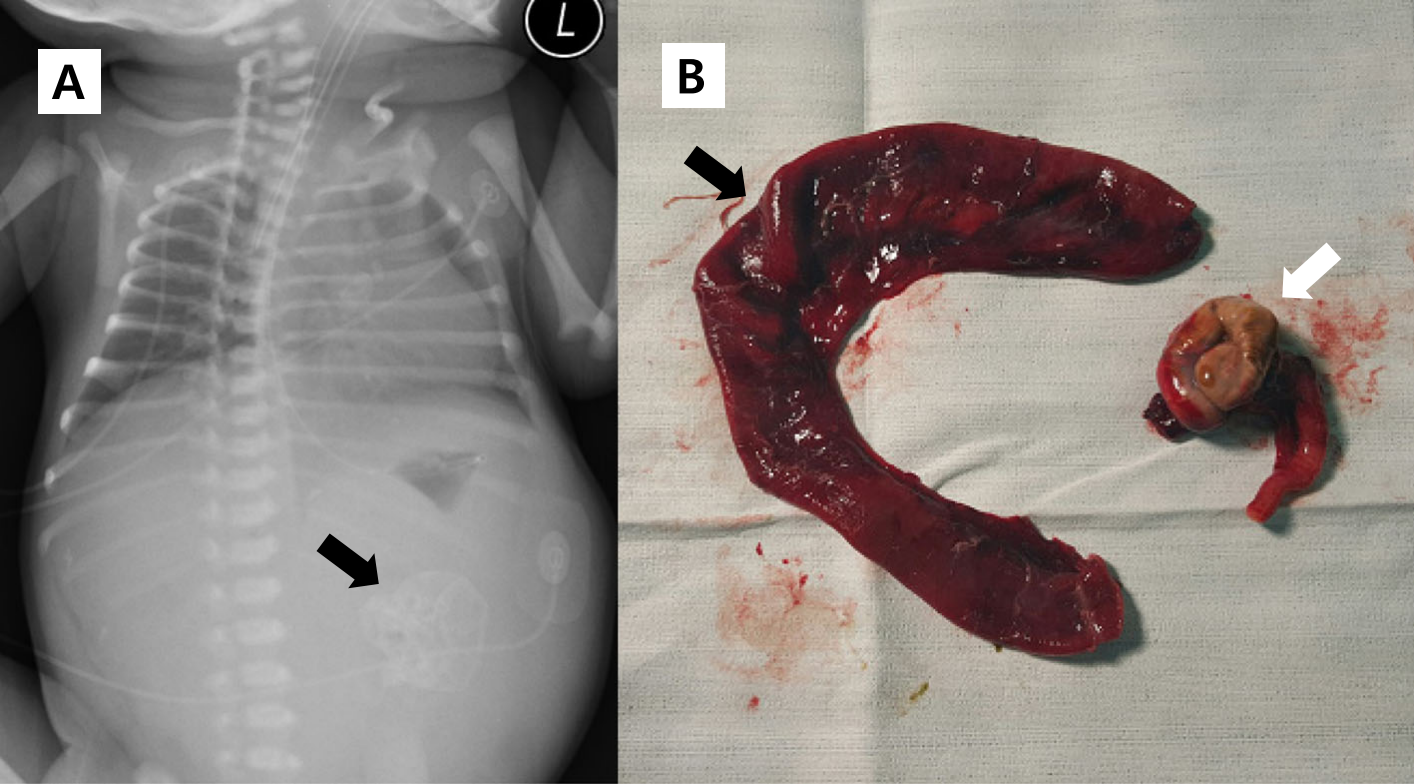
a A plain abdominal X-ray showing a calcified mass-like density in the left mid-abdomen (arrow). b A markedly distended proximal pouch in the ileum (arrow) and calcified distal pouch (white arrow), was resected
